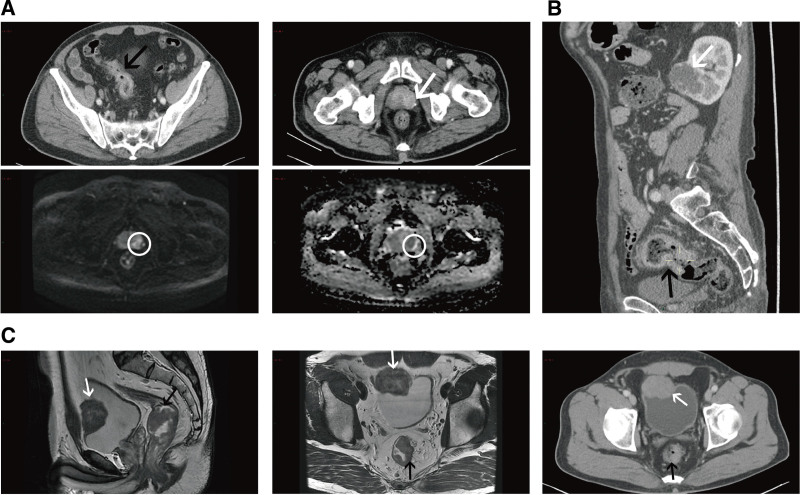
Urinary system incidentalomas included prostate, renal and bladder incidentalomas. (A) A 77-year-old man with sigmoid cancer (black arrow) and abnormal density shadow in the left peripheral zone of the prostate (white arrow and white circles) on CT and MR images. (B) A 73-year-old man with 5 cm sigmoid cancer (black arrow) and a 4.3 × 3.7 cm uneven-density mass (white arrows) in left kidney. (C) A 52-year-old man with rectal cancer (black arrow) and a 4.7 × 2.7 × 3.9 cm mass (white arrows) in bladder on median sagittal and transverse CT images.
